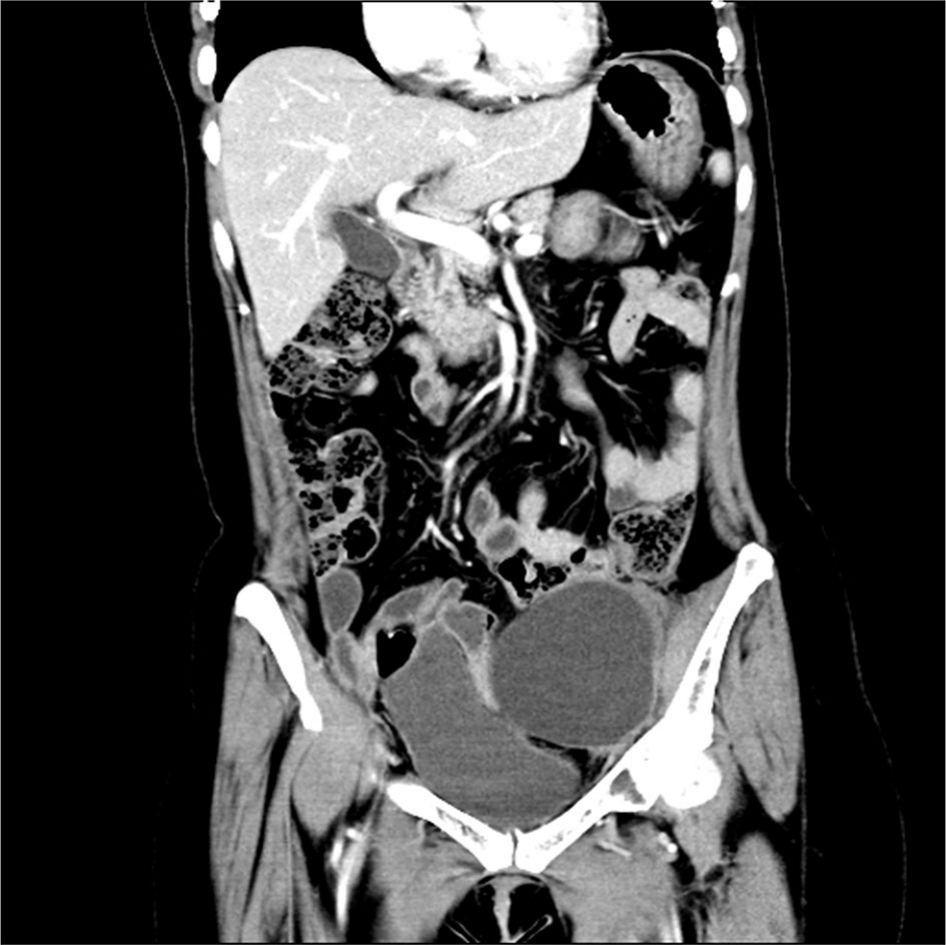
Pelvic MRI on June 5, 2020. No recurrence was found.
